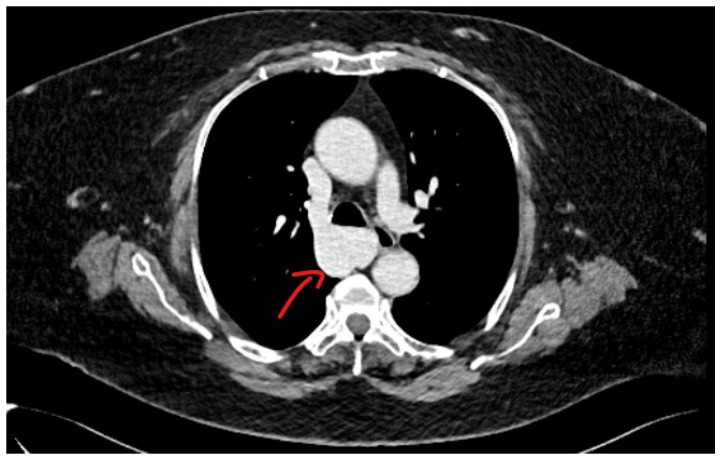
Chest angio-CT scan shows azygos ectasia (arrow).
